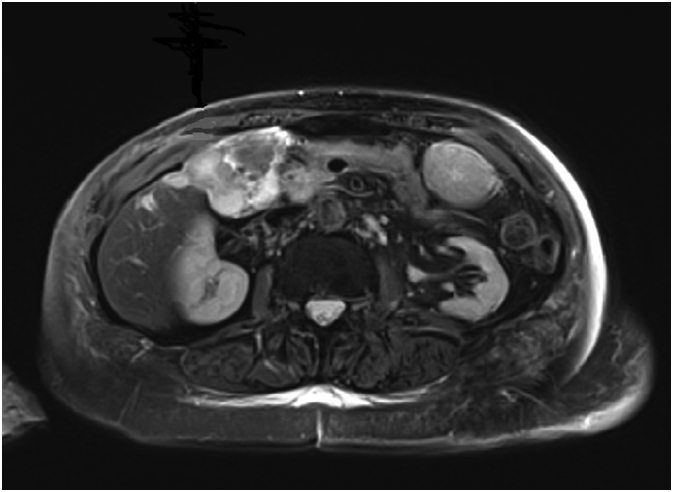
MRI with suspected gallbladder malignancy.
